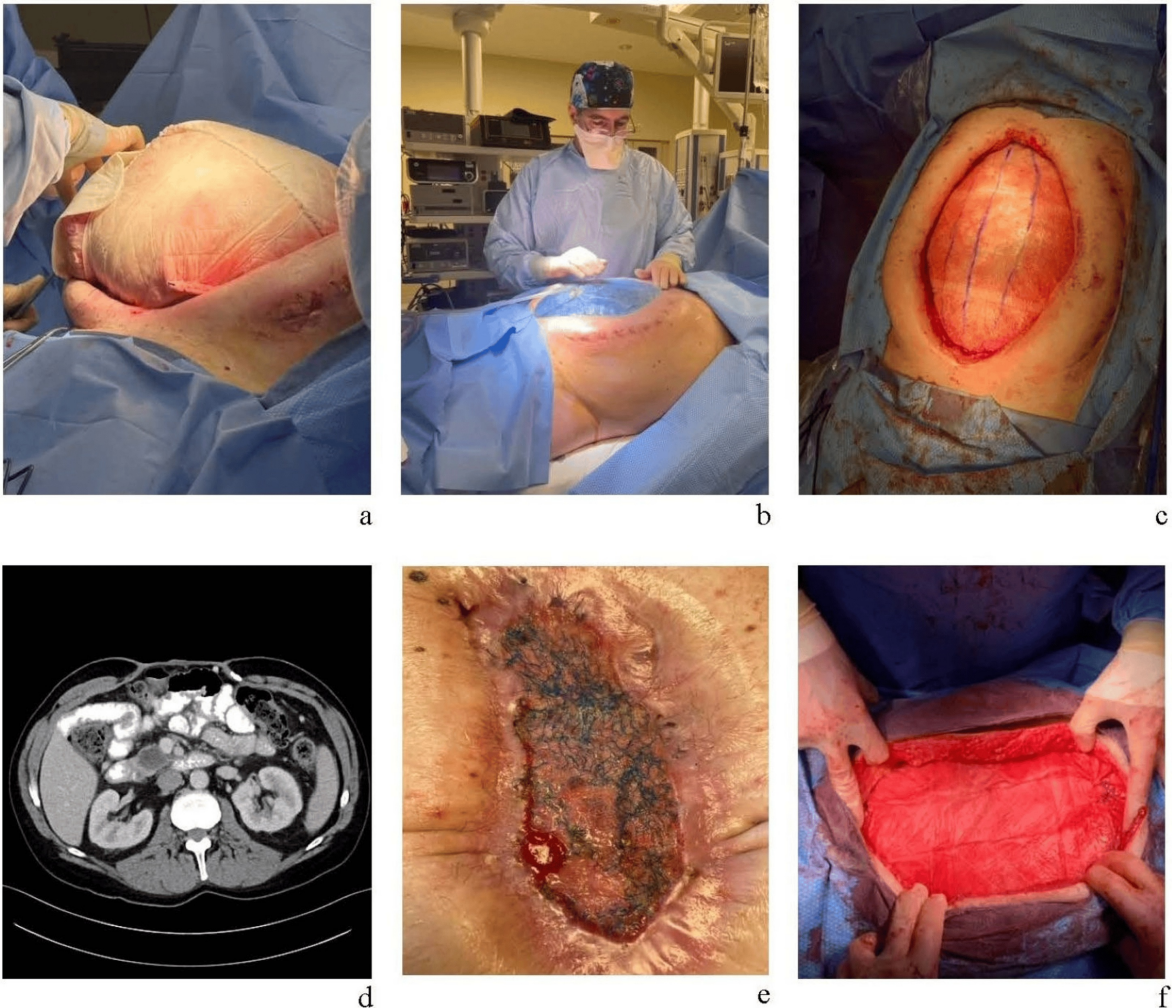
(a) Initial placement of OviTex® RTM. Three large OviTex® 2S RTMs sutured together; (b) Approximately two weeks post-RTM placement, significant decompression of the abdominal cavity can be seen. (c) After removal of previously placed OviTex® RTM and placement of a new OviTex® 1S RTM. The purple lines indicate midline and how close the wound edges could be approximated on either side. (d) CT scan showing ~14 cm diastasis of rectus muscles. (e) Granulation of RTM from intra-abdominal side. Note small bowel fistula in left lower aspect of the image. (f) Approximately 10 months post-initial RTM placement, showing the final RTM placement prior to complete closure of the defect. RTM: Reinforced tissue matrix; CT: computed tomography. Dr. David Baer is the surgeon performing the surgery as seen in figure (b).
